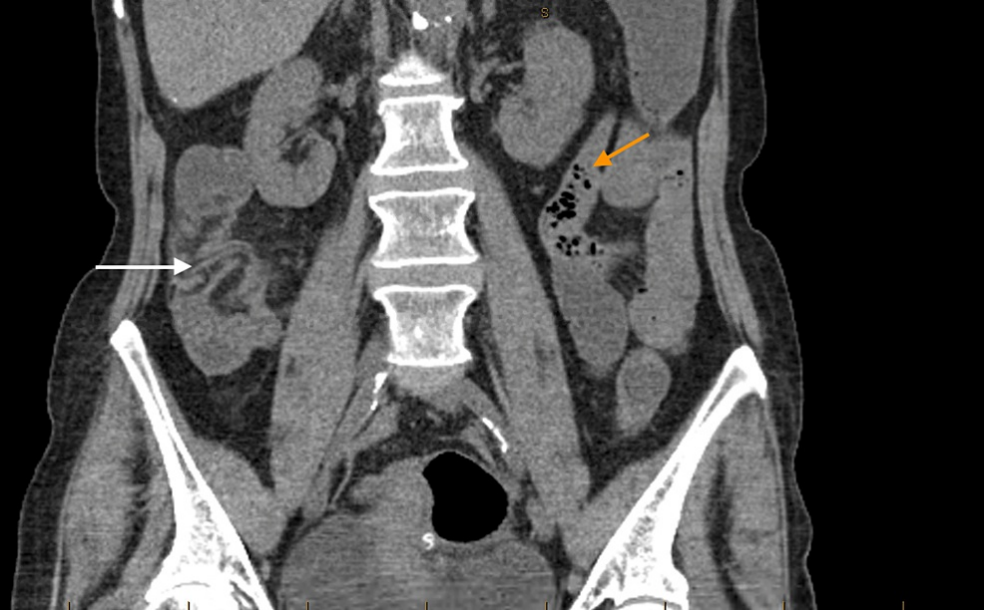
CT scan of abdomen and pelvis without oral contrast: colonic ileus with air fluid levels (shown with an orange arrow) along with colitis within the cecum and ascending colon (shown with a white arrow). CT, computed tomography
